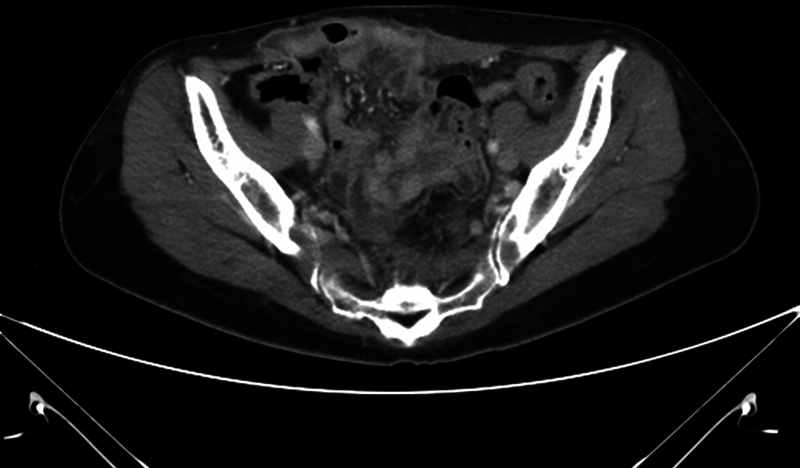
Axial contrast-enhanced CT image of case 2 showing abdominal wall hernia without overlying skin injury after HIFU therapy. Abdominal wall hernia with rectus abdominis muscle atrophy was noted. CT, computerized tomography; HIFU, high-intensity focused ultrasound.
